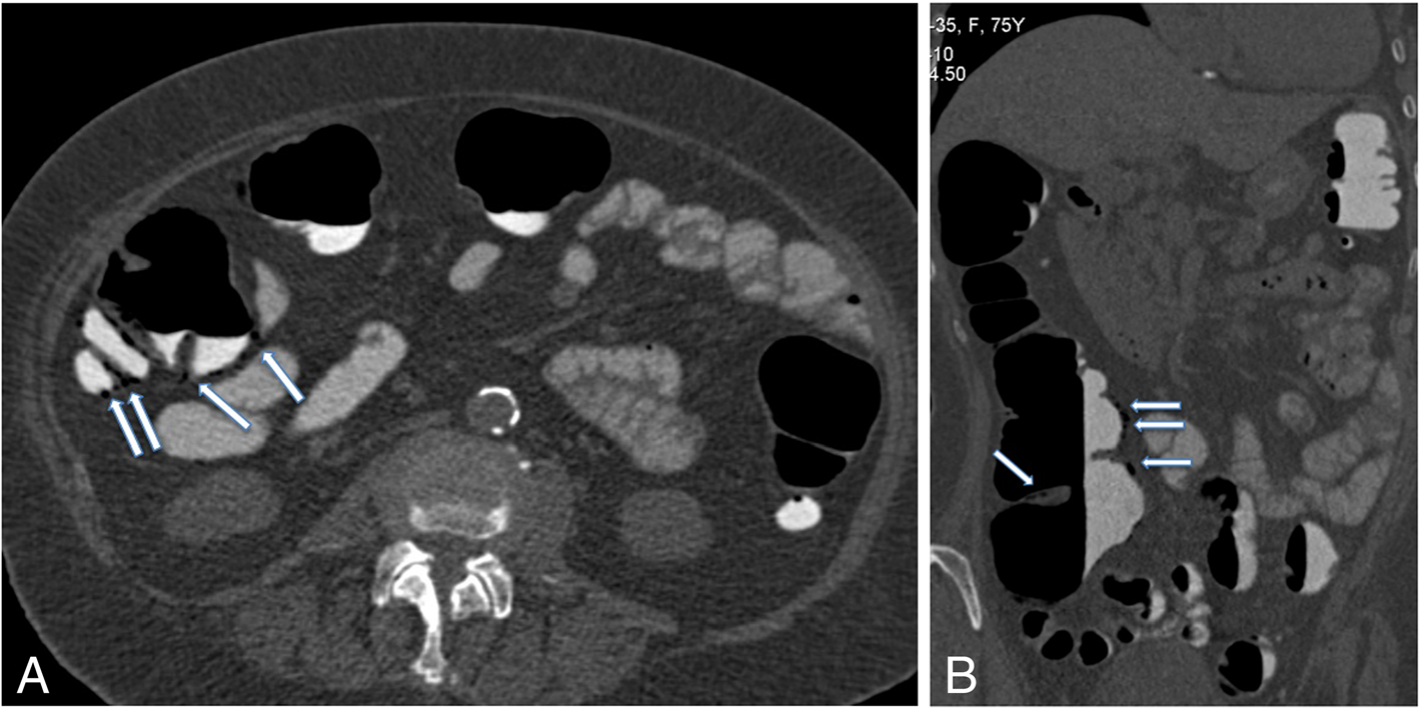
Two-dimensional computed tomography colonography images. (A) Supine axial and (B) right lateral coronal images show tiny curvilinear pneumatosis (arrows) involving the ascending colon, ileocecal valve and cecum without involvement of the transverse and left colon.
